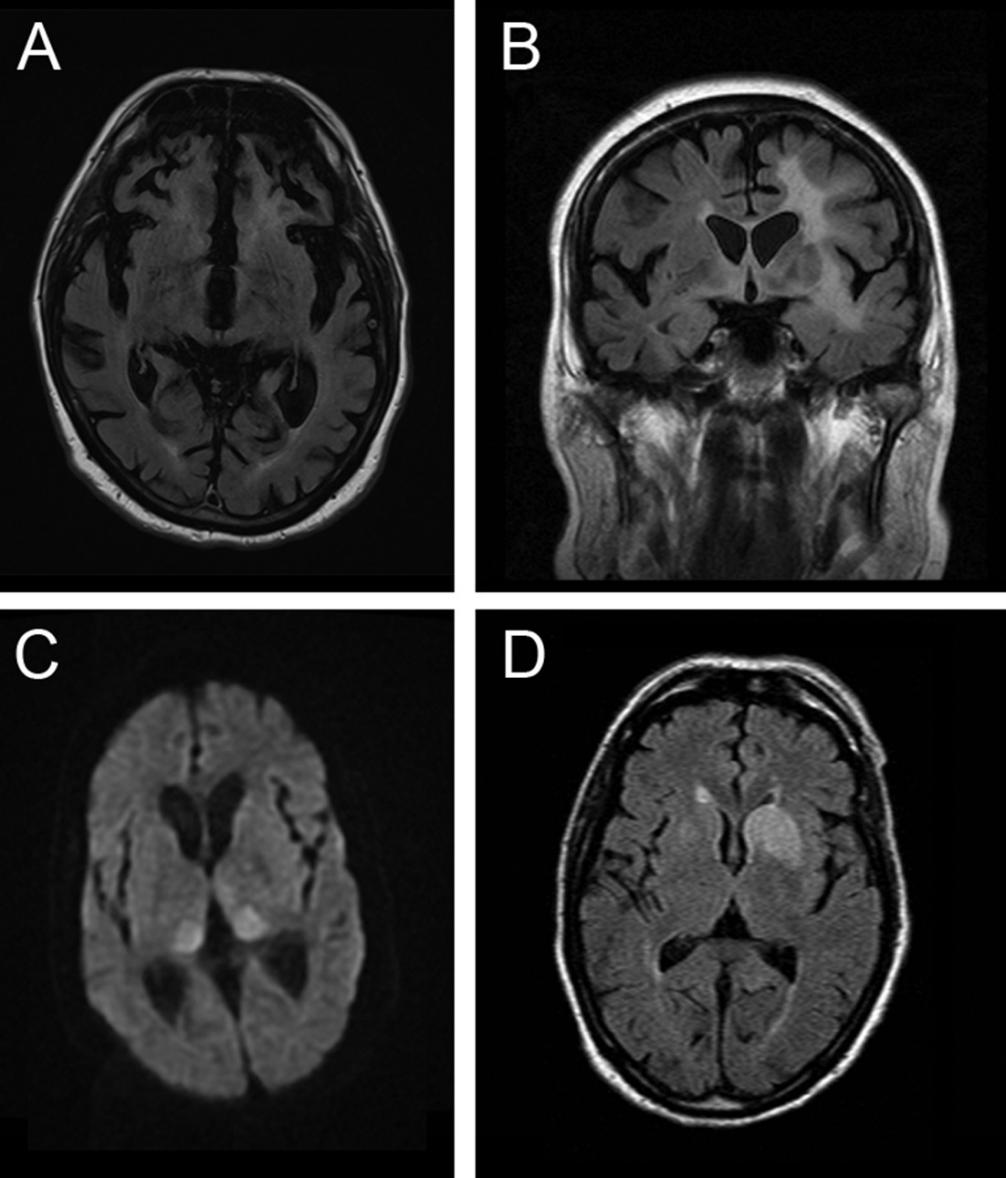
MR scan of brain in four patients presenting as mimics of prion disease. (A) *C9orf72* mutation showing severe generalised atrophy with no parenchymal abnormal signal. Severe atrophy does occur late in some cases of Creutzfeldt-Jakob disease (CJD), but usually diffusion-weighted sequences reveal restricted diffusion. (B) B-cell lymphoma confined at the time of presentation to the brain. Note the extensive white-matter signal change with the normal cortex. Occasionally, the leukoencephalopathic form of CJD has white-matter signal alteration, but this is associated with grey matter destruction. (C) *N*-methyl-D-aspartate antibody encephalitis with MR imaging showing the pulvinar sign. This sign develops in most cases of variant CJD and has rarely been described with other pathologies. (D) Voltage-gated potassium channel (Casp-1) antibody encephalitis showing unilateral basal ganglia high signal on diffusion-weighted imaging but not on the apparent diffusion coefficient map (scan not shown). Note that the swelling of the caudate is a feature that does not occur in CJD.
